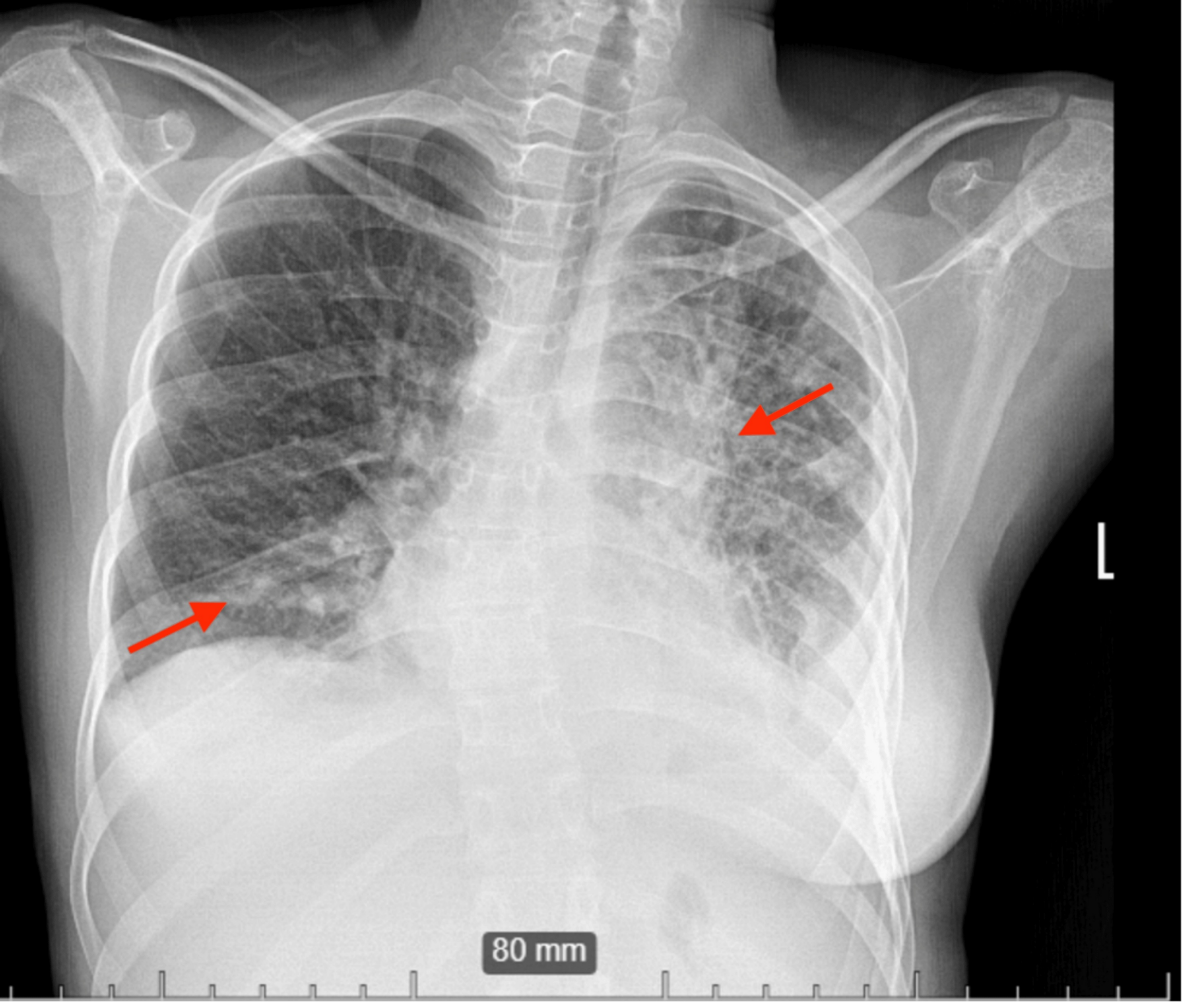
Chest X-ray showing left lung hypoplasia and bilateral interstitial infiltrates (red arrows).
